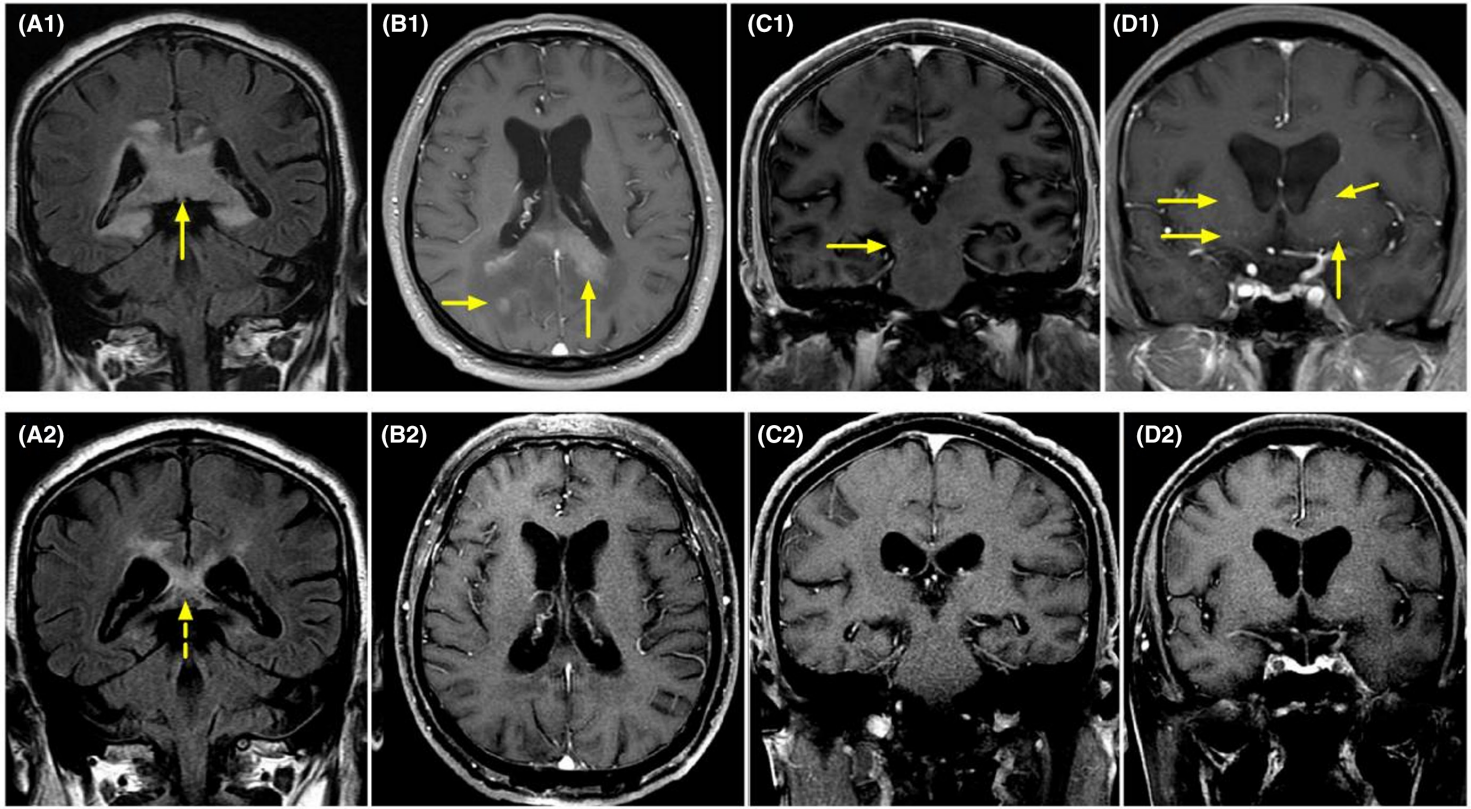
MRI features in a DNAP patient, Case 1. (A1) The T2‐abnormality is mainly located in the corpus callosum and has no obvious edema and mass effect. (A2) After intravenous methylprednisolone pulse therapy and mycophenolate mofetil treatment, the lesion is significantly reduced. (B1) Enhancement of lesions of the corpus callosum and occipital lobe. (C1) Outside the lesion, abnormal enhancement of the brainstem pia mater is observed (yellow arrow). (D1) Vascular enhancement is observed in the bilateral thalamus outside the lesion. (B2–D2) After treatment, no enhancement is observed in the parenchyma or pia mater.
